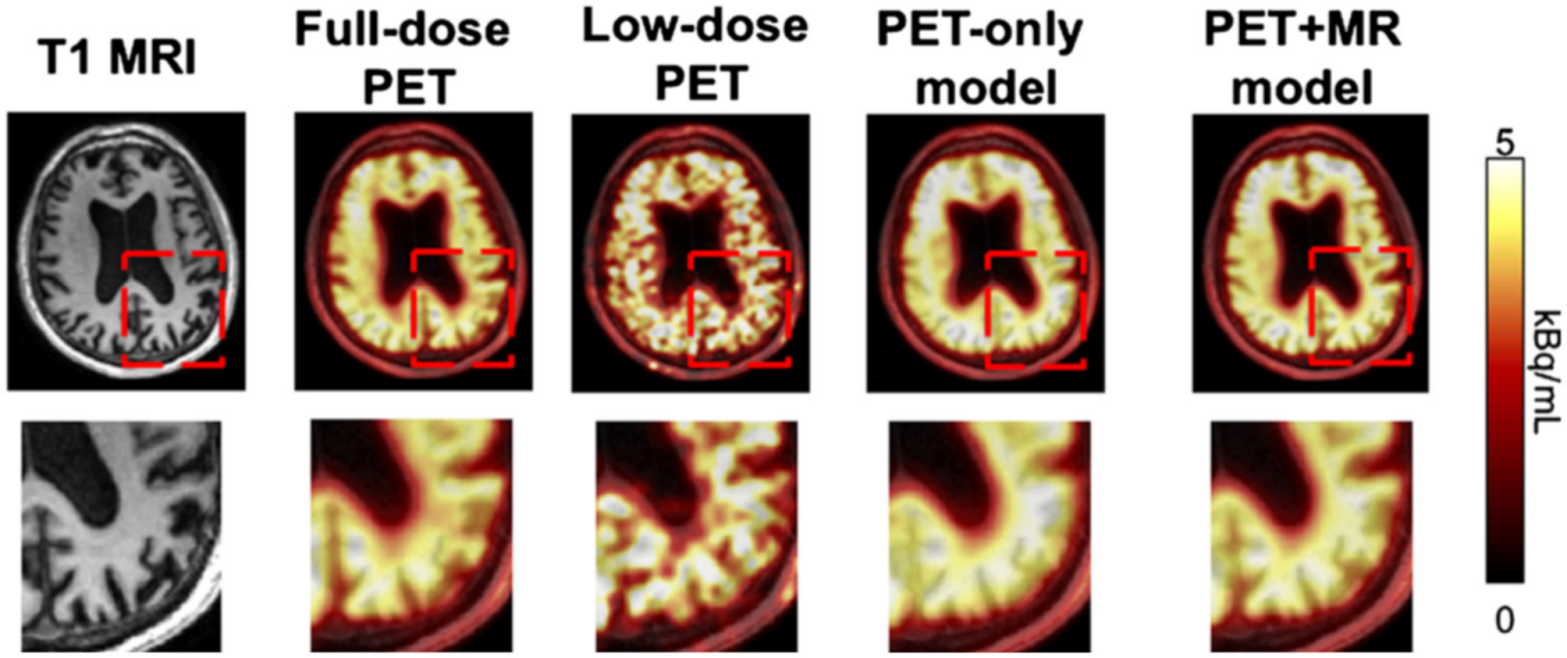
By training a CNN on simultaneously acquired MRI and PET data from PET/MRI, it is possible to generate an algorithm which can interpolate data in a low resolution low dose PET scan to generate more accurate imaging than would be produced by that same algorithm trained on only the PET data (22). Reprinted from Zaharchuk (40) with permission under the terms of the Creative Commons Attribution License, which permits unrestricted use, distribution, and reproduction in any medium, provided the original author and source are credited.
